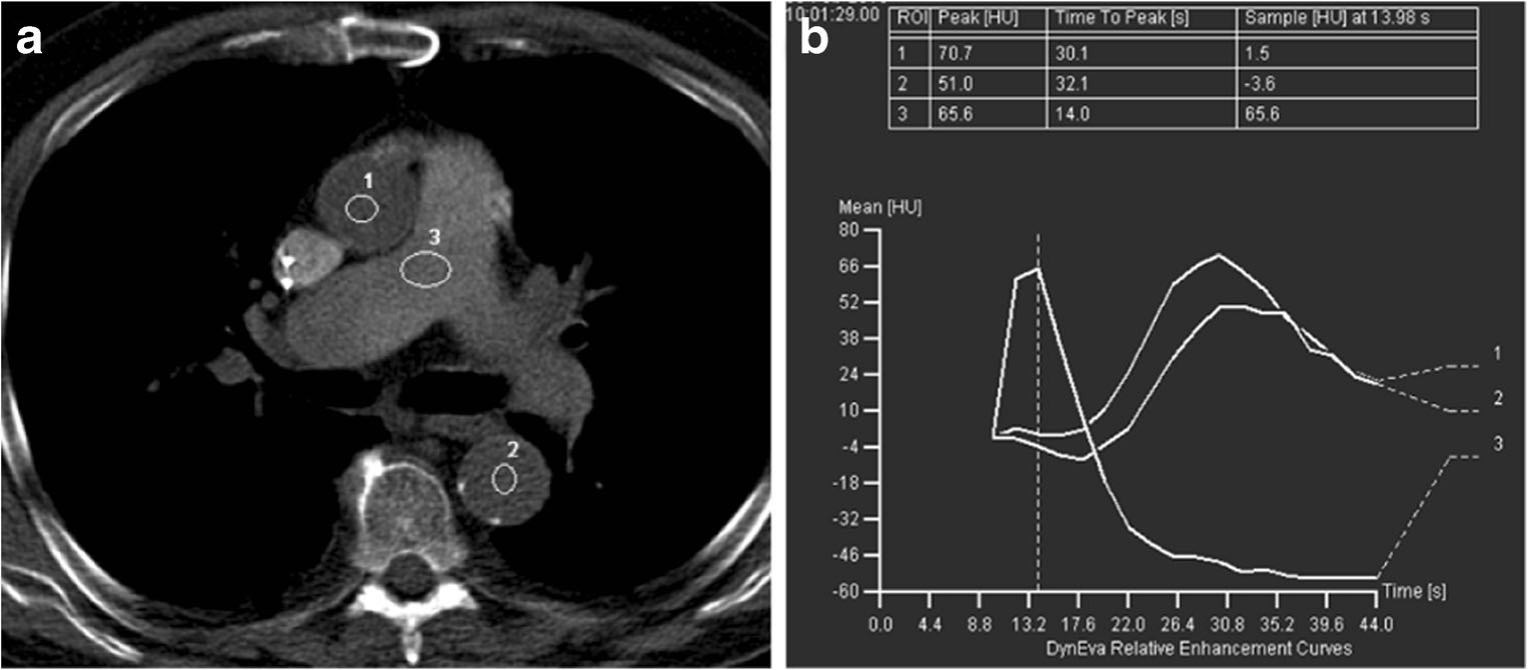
Test bolus technique for identification of contrast arrival in target vessel. Prolonged pulmonary circulation time in a patient with aortic stenosis. CT image during test bolus injection (a) with regions of interest over the ascending aorta (*1*), descending aorta (*2*) and pulmonary artery (*3*). Attenuation-time graph corresponding to the regions of interest (b) shows prolonged pulmonary circulation time of 16 s in this patient due to aortic stenosis (normal pulmonary transit time is approximately 7–9 s)
